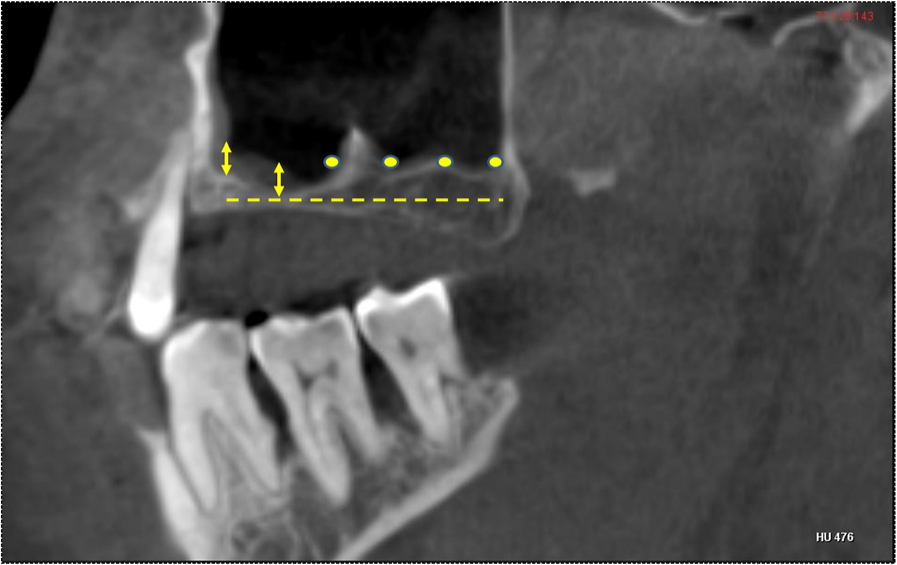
Sagittal CBCT section showing the points at which measurements were made in a edentulous study subject. Six equidistant points (arrows and dots) on a line (yellow discontinuous) connecting the anterior and posterior most points on the floor of the sinus in sagittal section. (Dots indicate areas with no MT)
